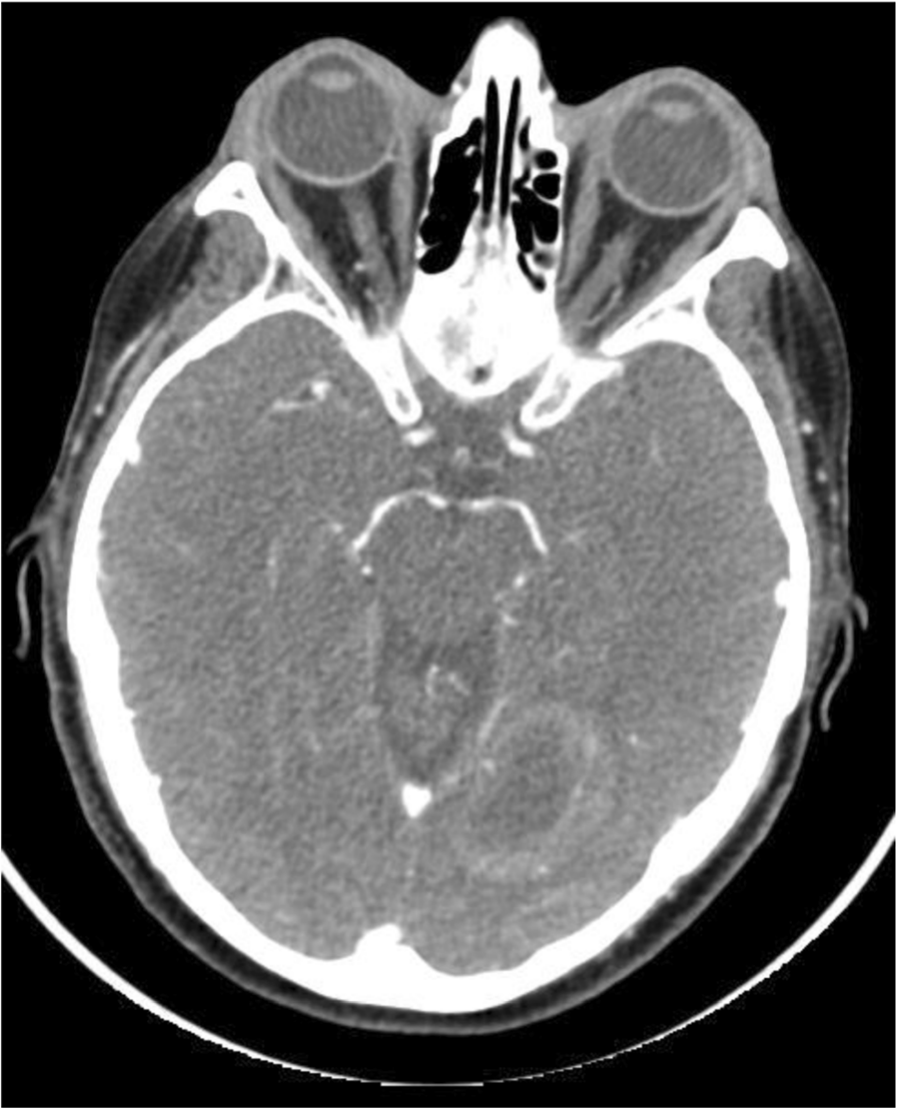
CT Scan of the space occupying lesion found in the left occipital Lobe of the brain
